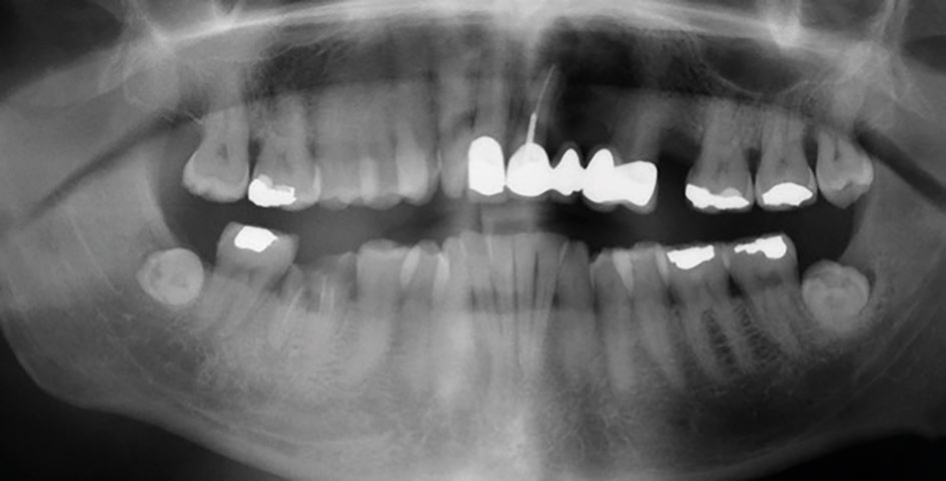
Dental panoramic radiograph of a patient before radiotherapy for head and neck cancer
